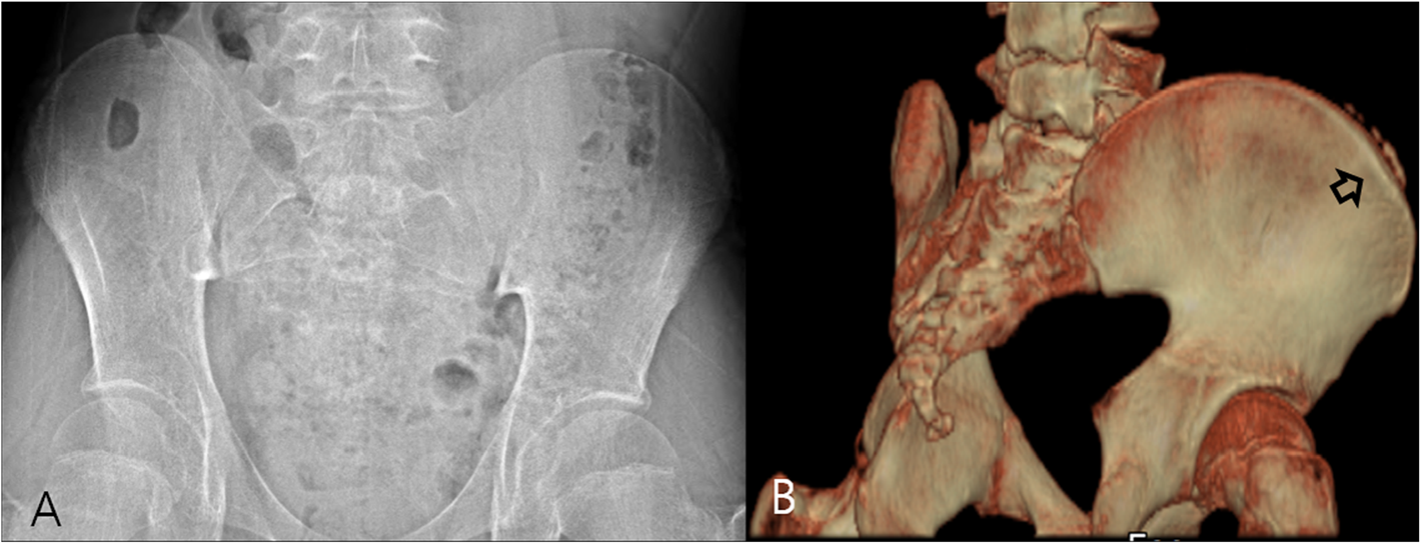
Micro-ossification. (A) Plain radiography performed on a 12-year-old boy did not reveal excursion of the apophysis and was graded as Risser stage 0. (B) A 3D-CT image of the same patient revealed micro-ossification of the iliac apophysis and was graded as Risser stage 1. The lower resolution and sensitivity of PR failed to show isolated non-linear ossification.
